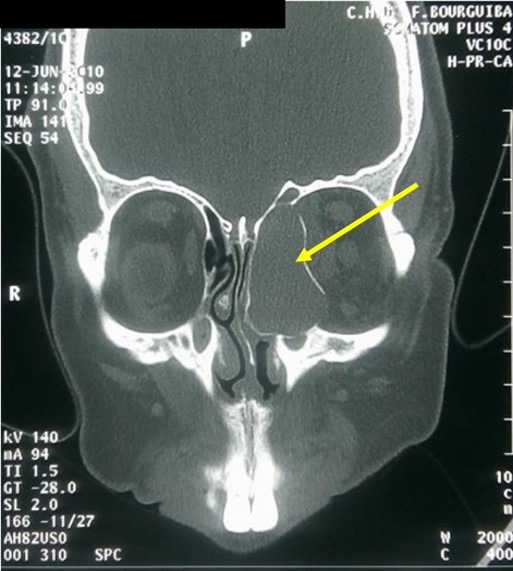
CT scan of the facial mass in the bony window, coronal section: left ethmoid mucocele with orbital extension (thinning of the bony walls and rupture in places)
